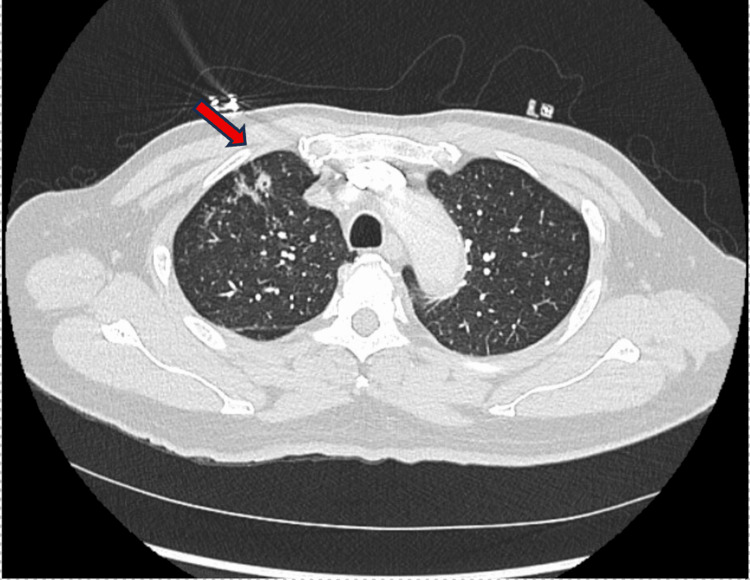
Cavitary pulmonary lesion concerning for pulmonary tuberculosis Computerized tomography angiography (CTA) chest revealed a 1 cm nodular lesion with central cavitation located in the right upper lobe of the lung (red arrow).
